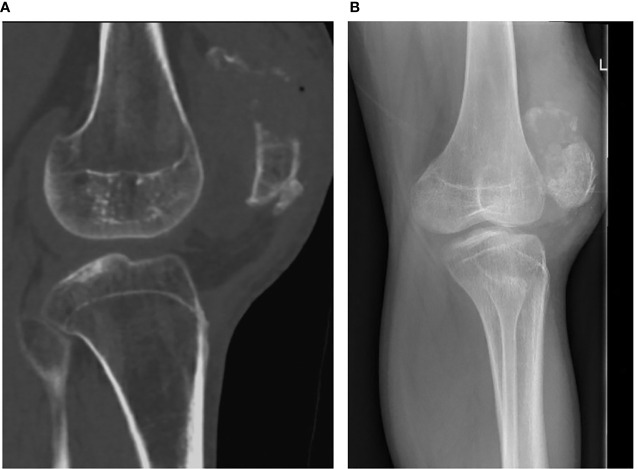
Sagittal CT view (A) and lateral radiograph (B) of the left patella showing an avulsion fracture of the superior patella pole.
